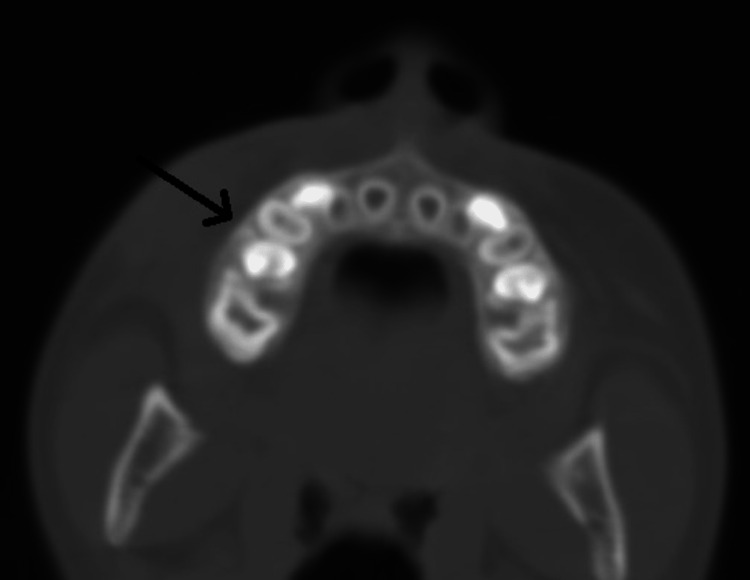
Enhanced CT scan of the face showing an intact underlying cortical bone (arrow)
